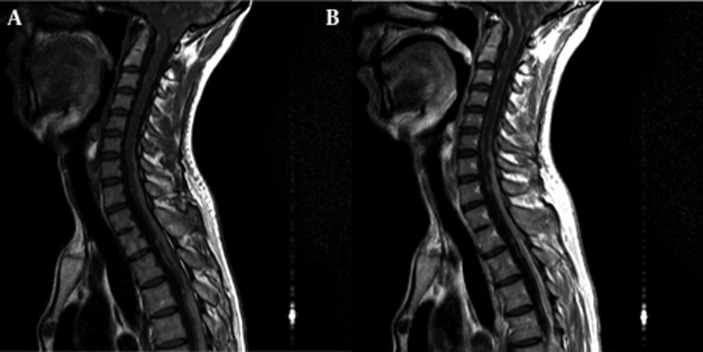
A, Mid-sagittal T1-weighted image through the cervical and upper thoracic region is remarkable for hypersignal leptomeningeal lesions of melanocytoma as a result of T1 shortening effect of their melanin content; B, Mid-sagittal contrast enhanced T1-weighted image is brought in the corresponding level, revealing contrast enhancement in the aforementioned lesions.
